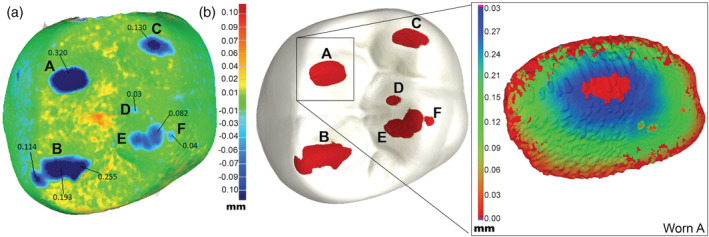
(a) Top view of superimposed baseline scan and worn scan from intraoral scanner using metrology software. Blue areas show areas of wear according to color scale on the right of the image; (b) Top view of a Dentoform model showing wear facets (red) obtained using micro‐CT measurements and representative wall thickness analysis obtained using VGStudio Max 3.0 for worn area A
